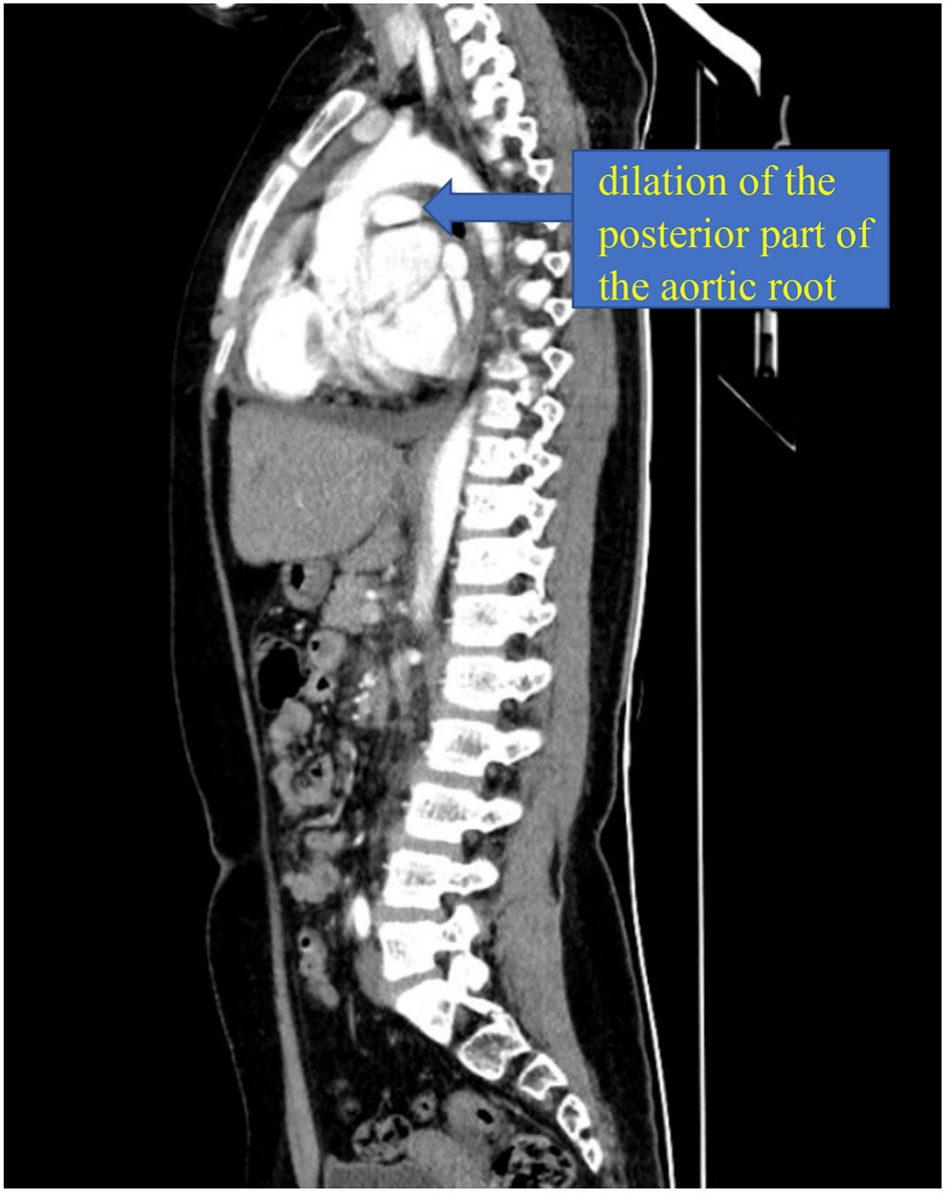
Computed tomography (CT).
